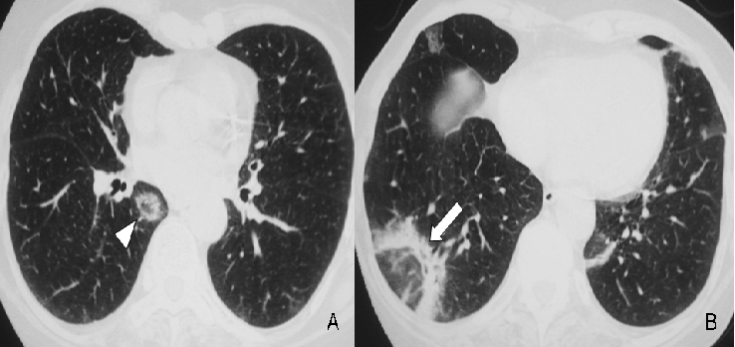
High-resolution computed tomography (HRCT) at presentation at the level of the lower pulmonary veins (A) and lower lobes (B) at lung windowing shows a ring-like opacity (arrowhead) and subpleural band-like opacity (arrow) with ground-glass in the centre of them consistent with the "reversed halo sign".
